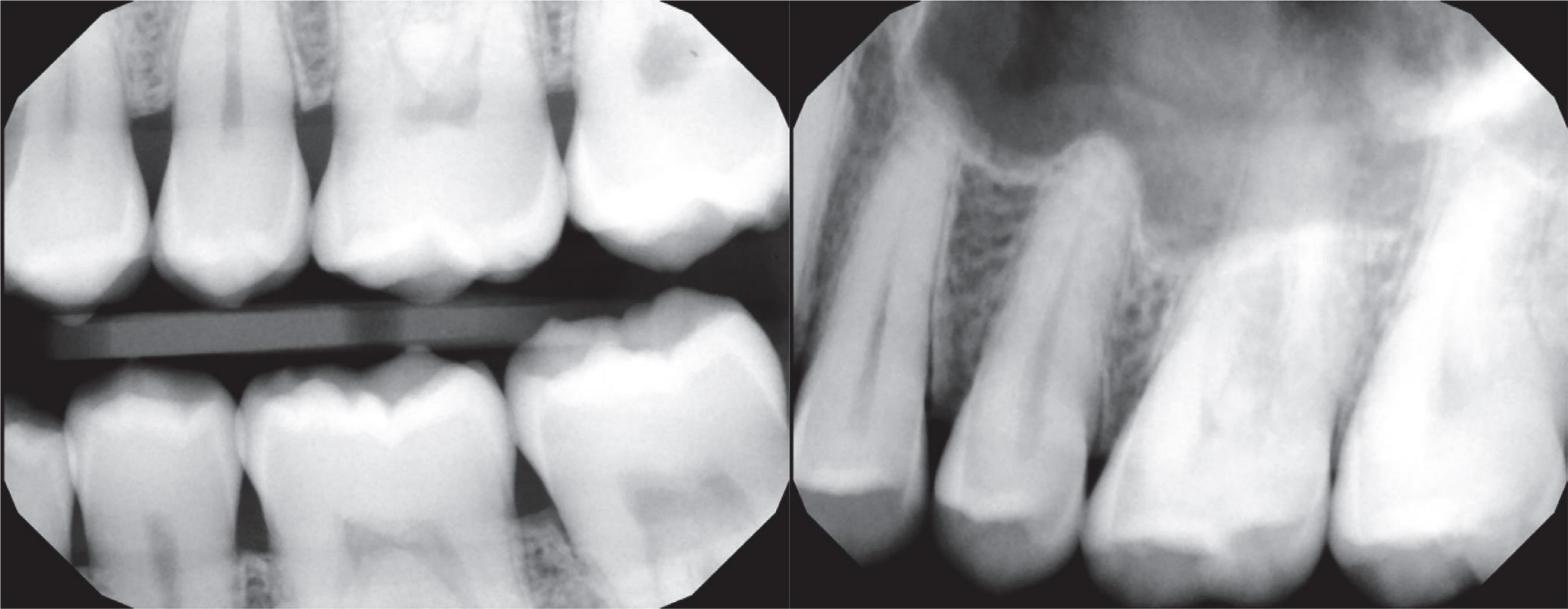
Upper left bitewing + periapical radiographs.
